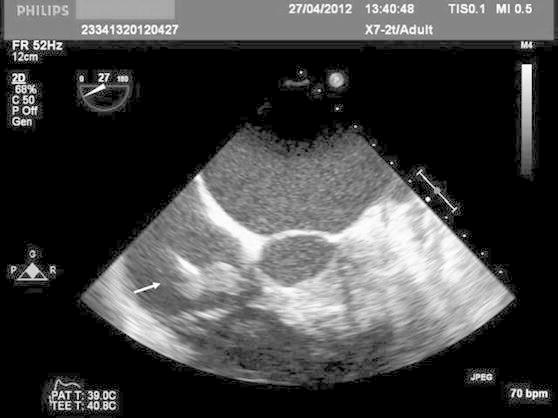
A mass in the right atrium (indicated by arrow), above the tricuspid valve, attached to the device lead on the transesophageal echocardiography (TEE)
